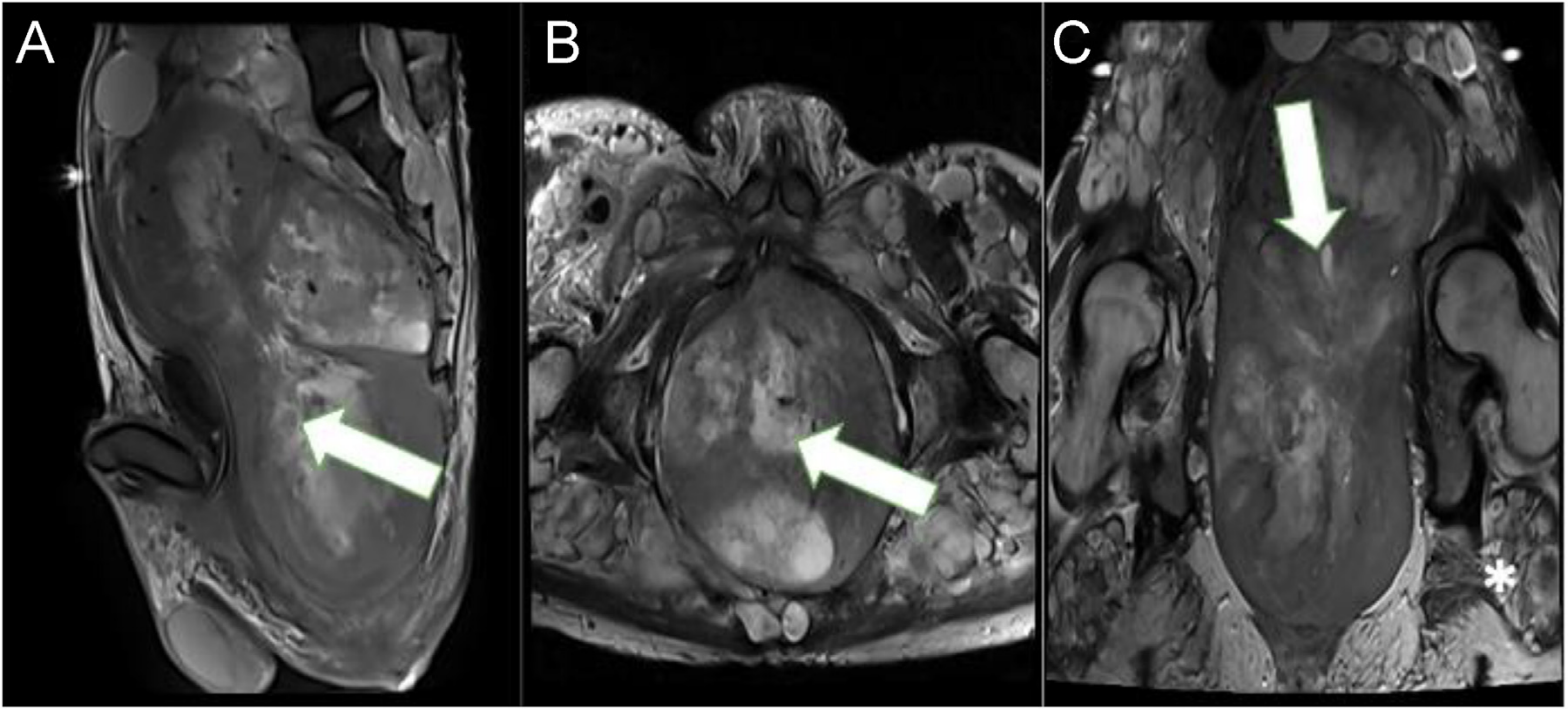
(A) Sagittal, (B) axial, and (C) coronal view of T2-weighted abdominopelvic MRI = revealed heterogenous mass from the prostate extending to the abdominal cavity (A-C, white arrow). There were masses with target signs in the presacral space, along with intra and intermuscular (*).
